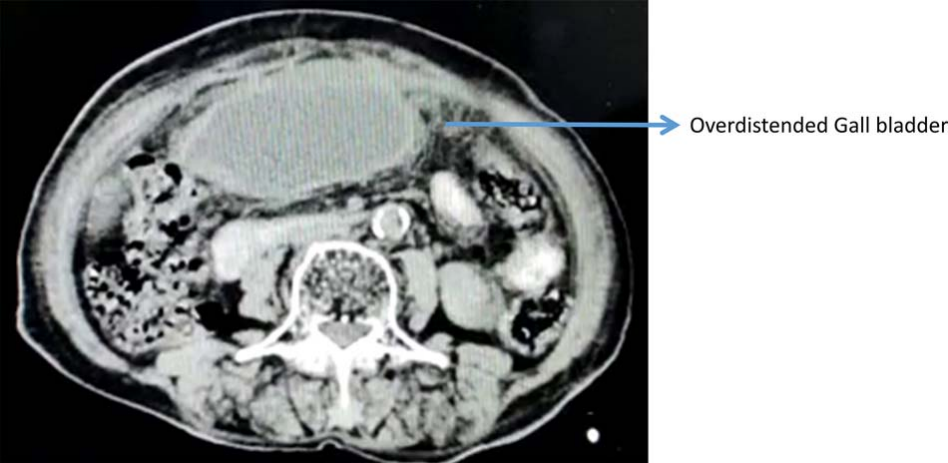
Ultrasonography of abdomen showing over distended GB.
